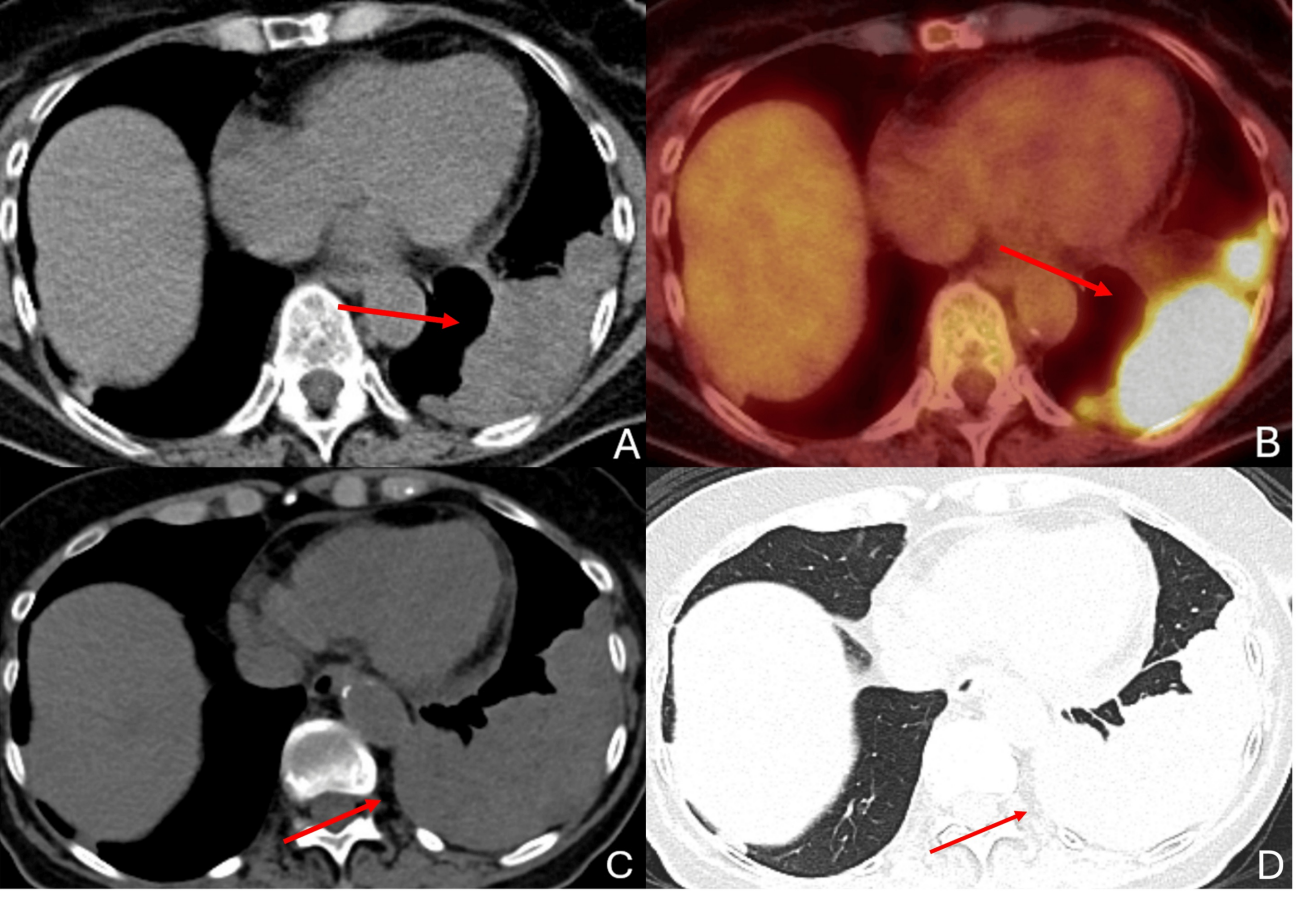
Diagnostic Computed Tomography Imaging of a Bulky Pleural-Based Mass Positron emission tomography-computed tomography (PET/CT) scan three months prior to treatment showing the transverse dimension on CT (A) with PET overlay (B) of the pleural-based mass extending into the upper abdomen. The mass measures 8.5 x 4.4 centimeters (cm) in the transverse dimension with a maximum standardized uptake value (SUV) of 16.5. There was evidence of progression seen on the non-contrast CT scan one week prior to treatment, measuring 11.4 x 5.3 cm in the transverse dimension and seen on mediastinal (C) and lung (D) windows. Red arrows indicate the left lower lobe (LLL) mass.
